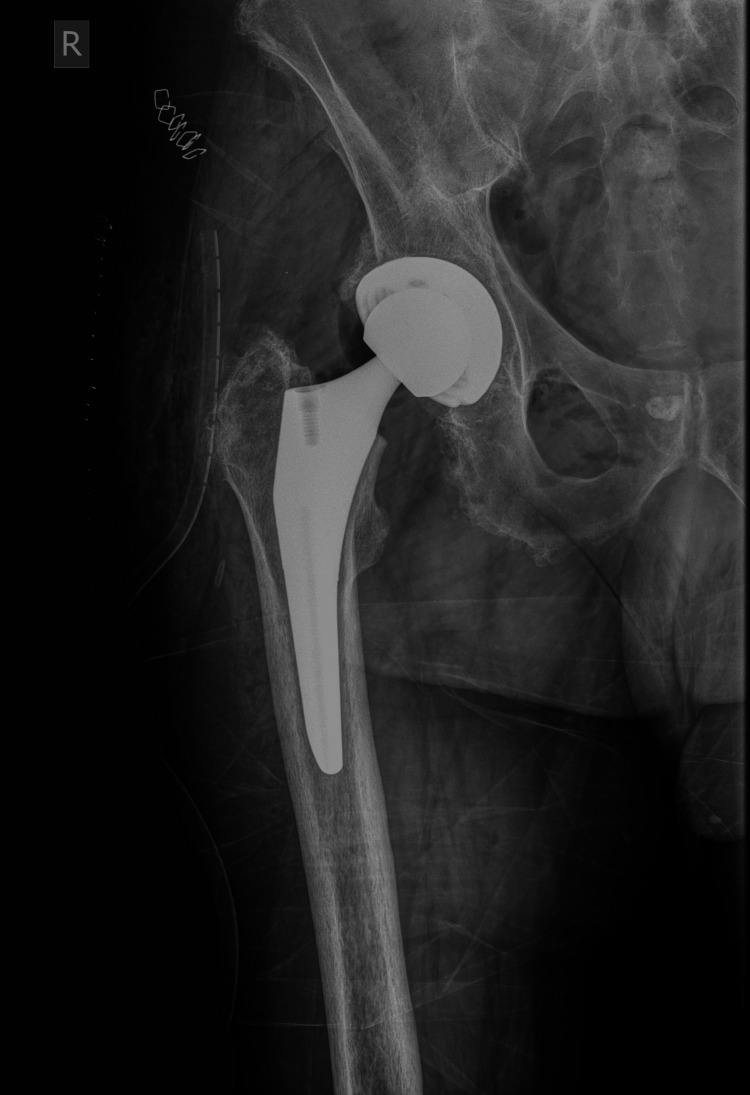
Postoperative radiograph of the right hip in AP view AP: anteroposterior
